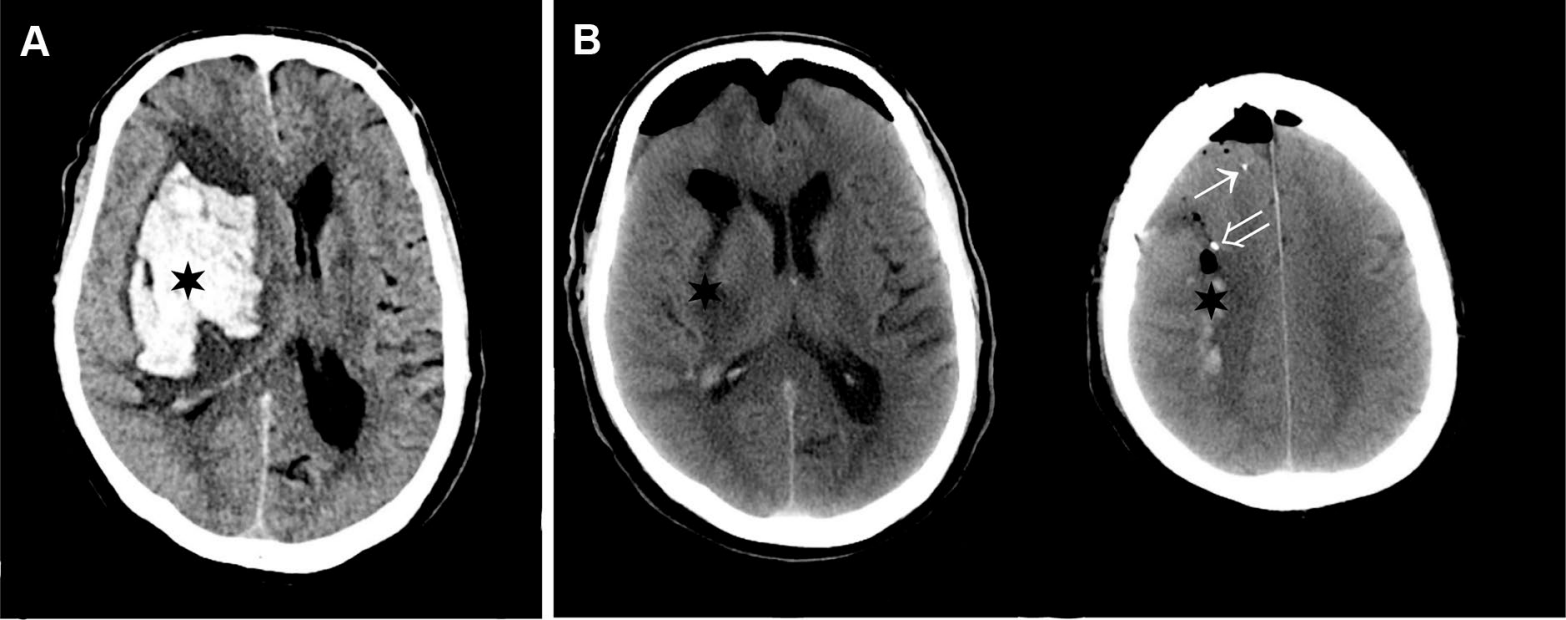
Preoperative and postoperative CT scan. a, Preoperative CT scan of a 68-year-old man presenting with an intracerebral hemorrhage (ICH) (black star) in the right basal ganglia. b, Following surgical evacuation of the ICH, a postoperative CT scan shows the hematoma cavity (black star) and the tip of the microdialysis (MD) catheters placed in the perihemorragic zone (PHZ) open arrow and seemingly normal cortex (SNX) (closed arrow), respectively. CT= computed tomography
